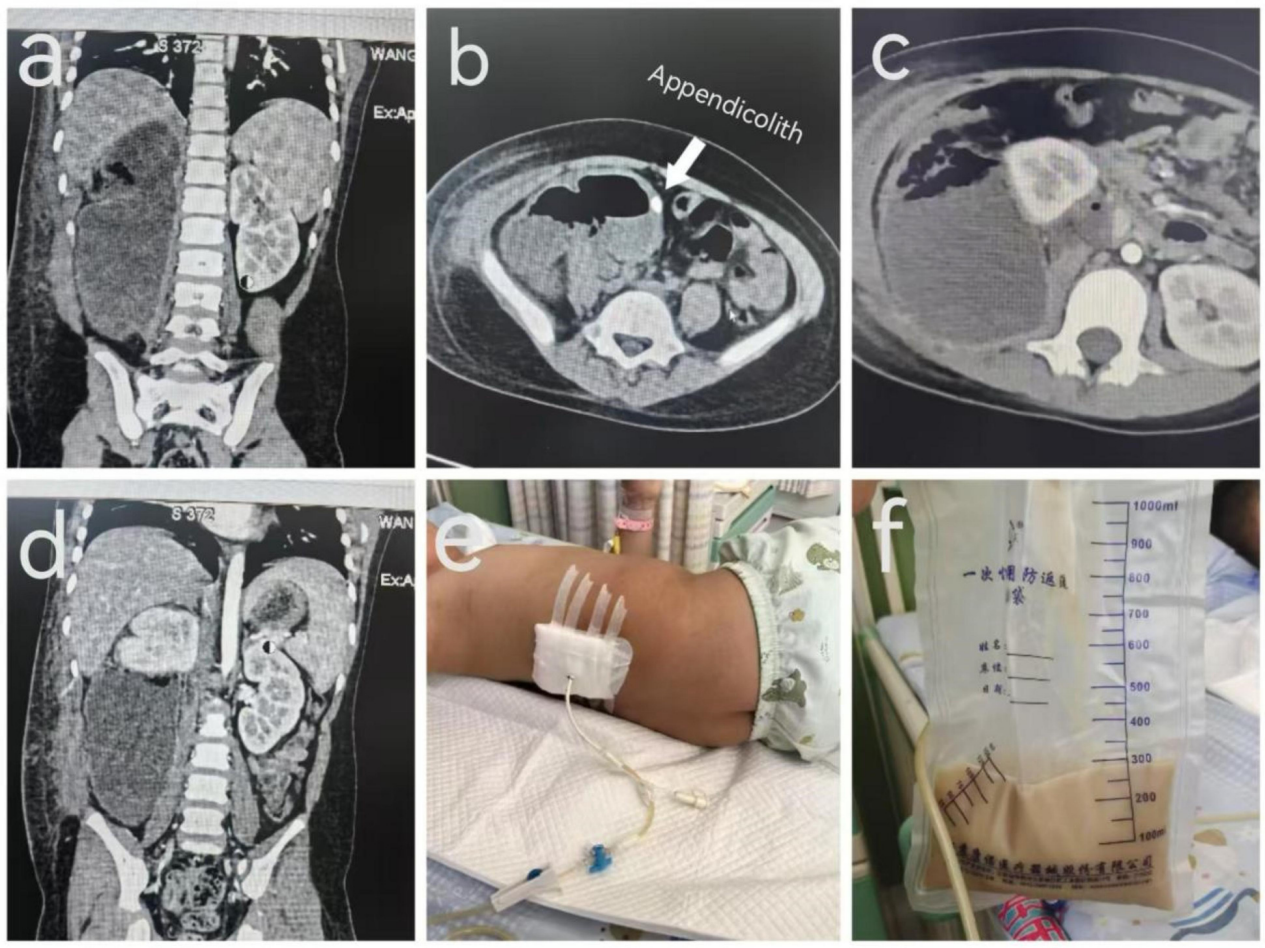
Preoperative CT nnnand intraoperative drainage images of a 5-year-old boy with a retroperitoneal appendiceal abscess. [(a) Preoperative CT image showing the maximal extent of the abscess. (b) Appendicolith. (c) Displaced kidney. (d) Compressed kidney. (e) Catheter drainage placement. (f) Drained purulent fluid].
